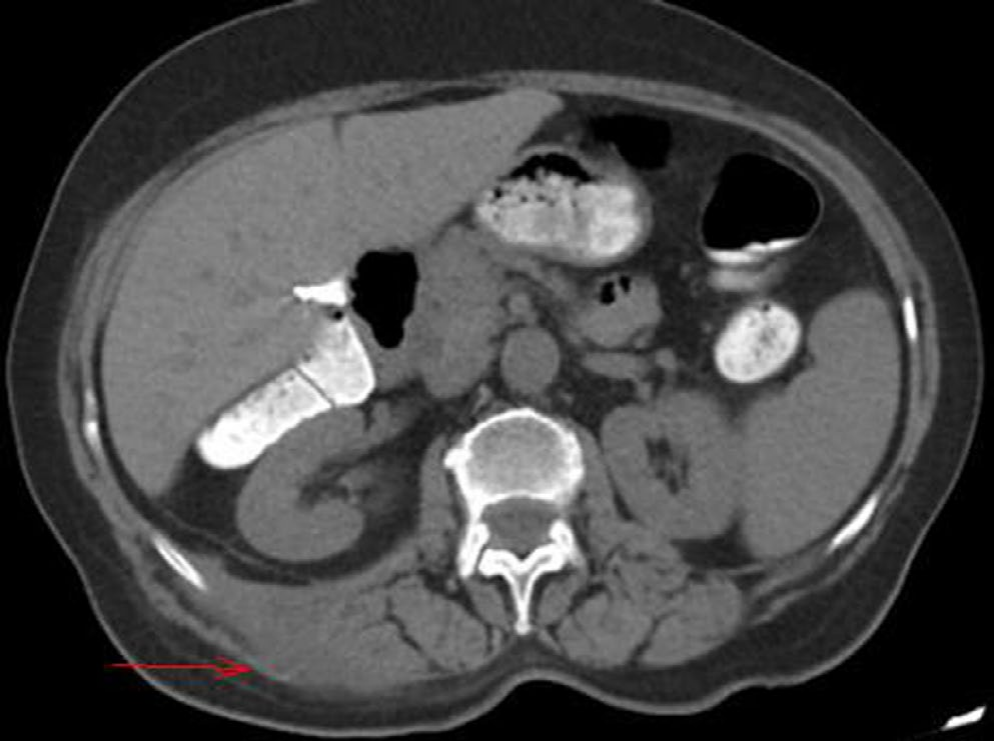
CT revealed the presence of abscess formation (red arrow) at the superficial and intermediate muscle layer of the posterior abdominal wall (latissimus dorsi, external oblique and internal oblique muscle) without involvement of the deep muscle layer (quadratus lumborum and psoas muscle)
